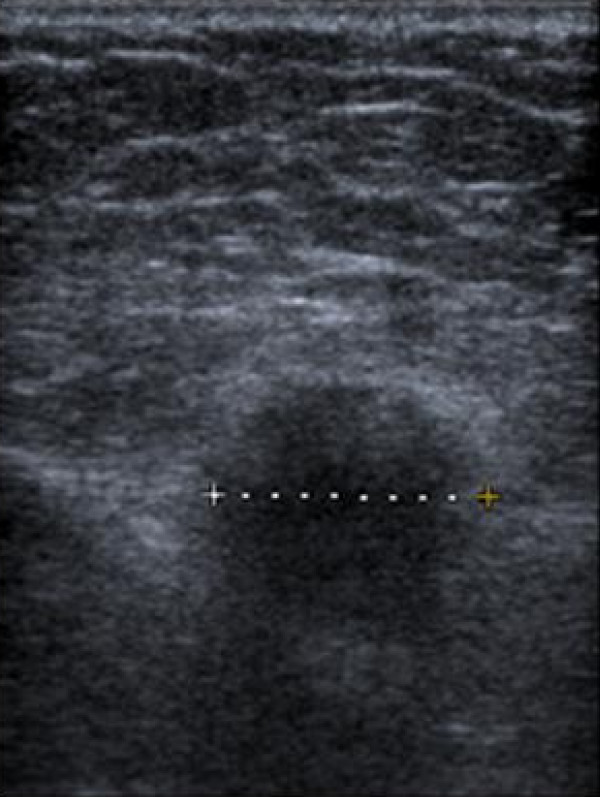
Sonographic appearance of granular cell tumour. Ultrasound shows an ill defined hypoechoic mass with echogenic haloing. It is difficult to differentiate this mass from a primary breast lesion, based on the sonographic findings.
